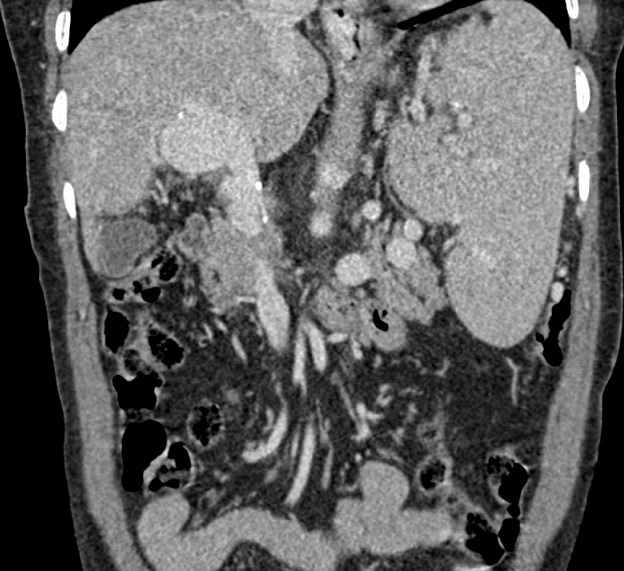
Coronal reconstruction of a portal-phase angio-CT showing portal aneurysm, thin wall calcifications appear in the portal trunk and the aneurysm
